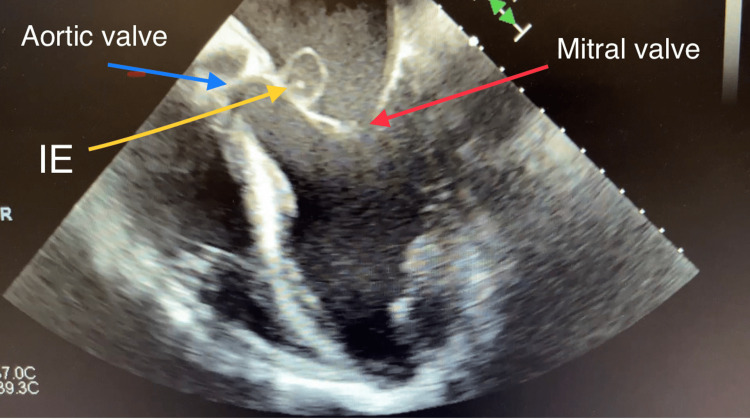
Transesophageal Echocardiogram Showing Infective Endocarditis of Mitral Valve and Aortic Valve The TEE shows extensive endocarditis eroding the aortomitral curtain with a fistula forming between the left ventricle and left atrium.
